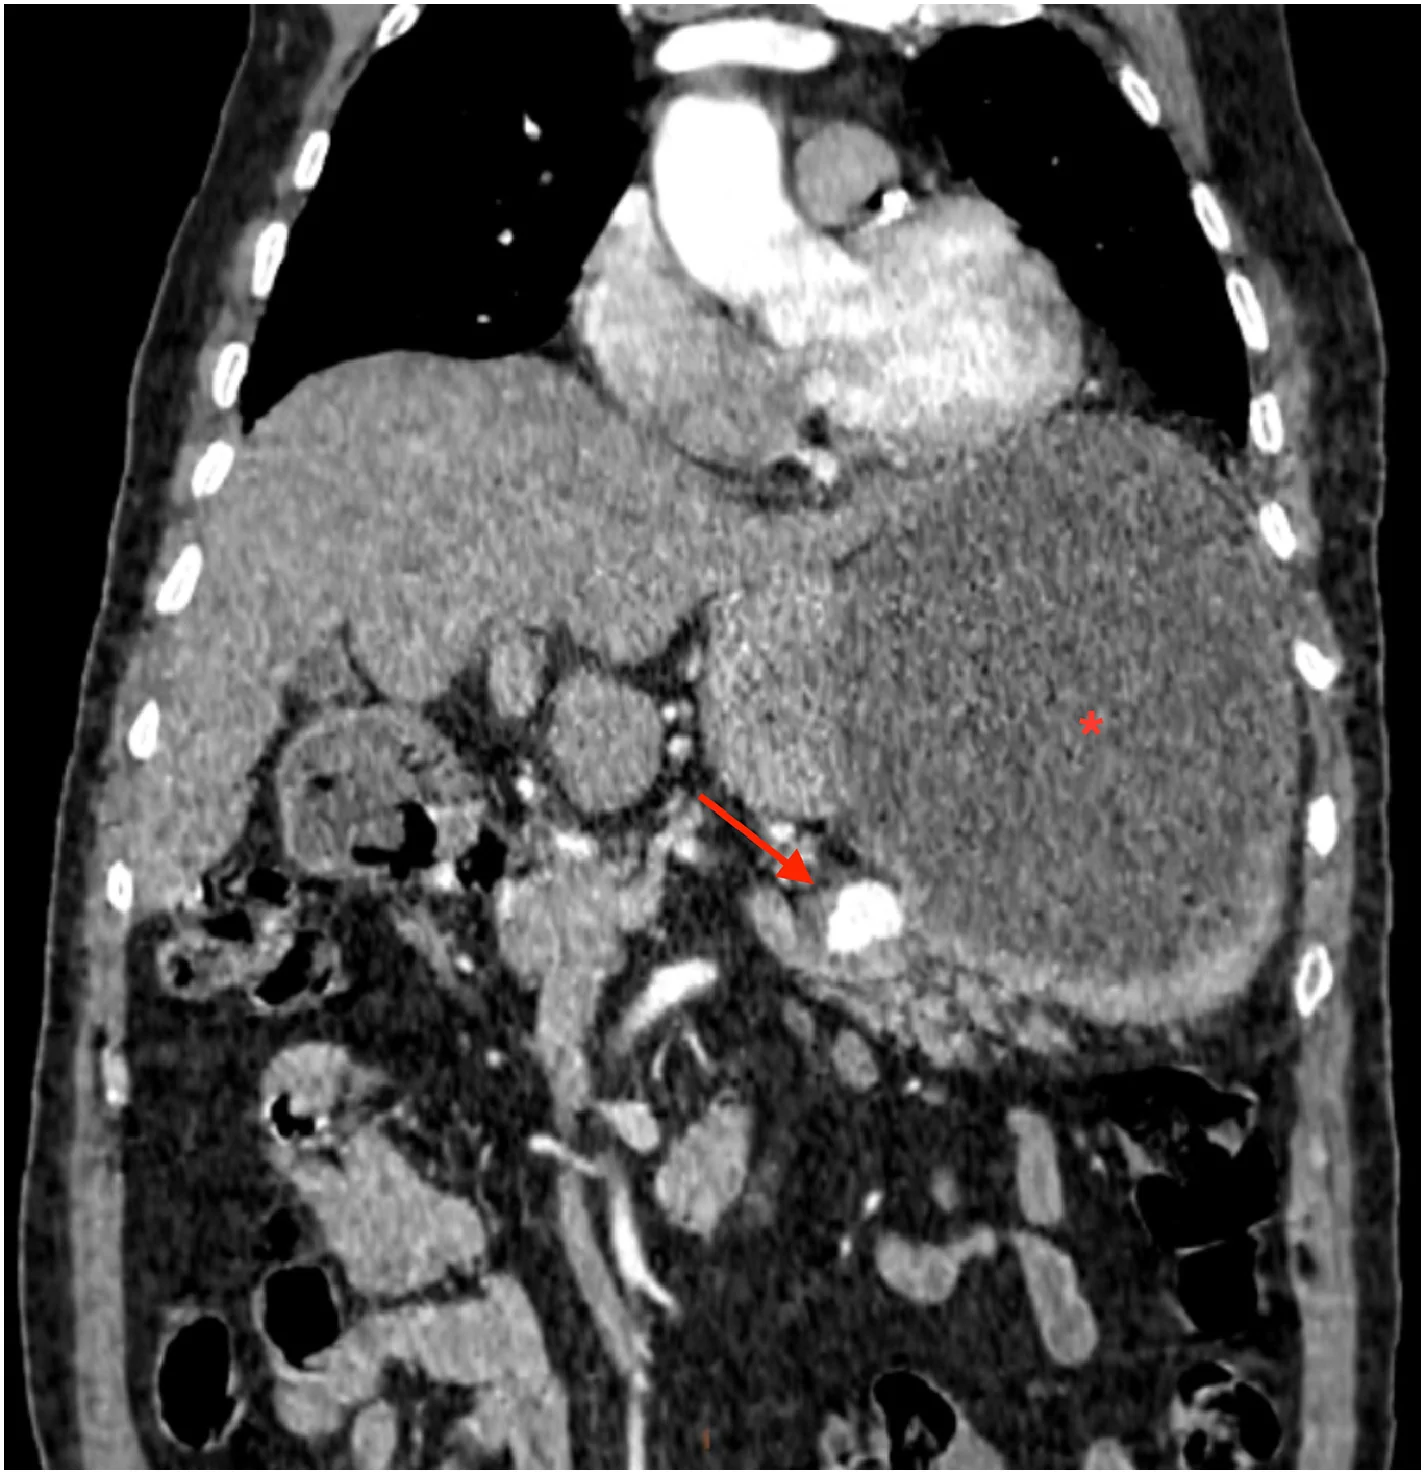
Coronal contrast-enhanced CT reconstruction, portal venous phase, demonstrating the relationship between the large cystic splenic lesion (asterisk) and adjacent abdominal structures. Arrow indicates the inflammatory/vascular component, including the suspected pseudoaneurysm.
